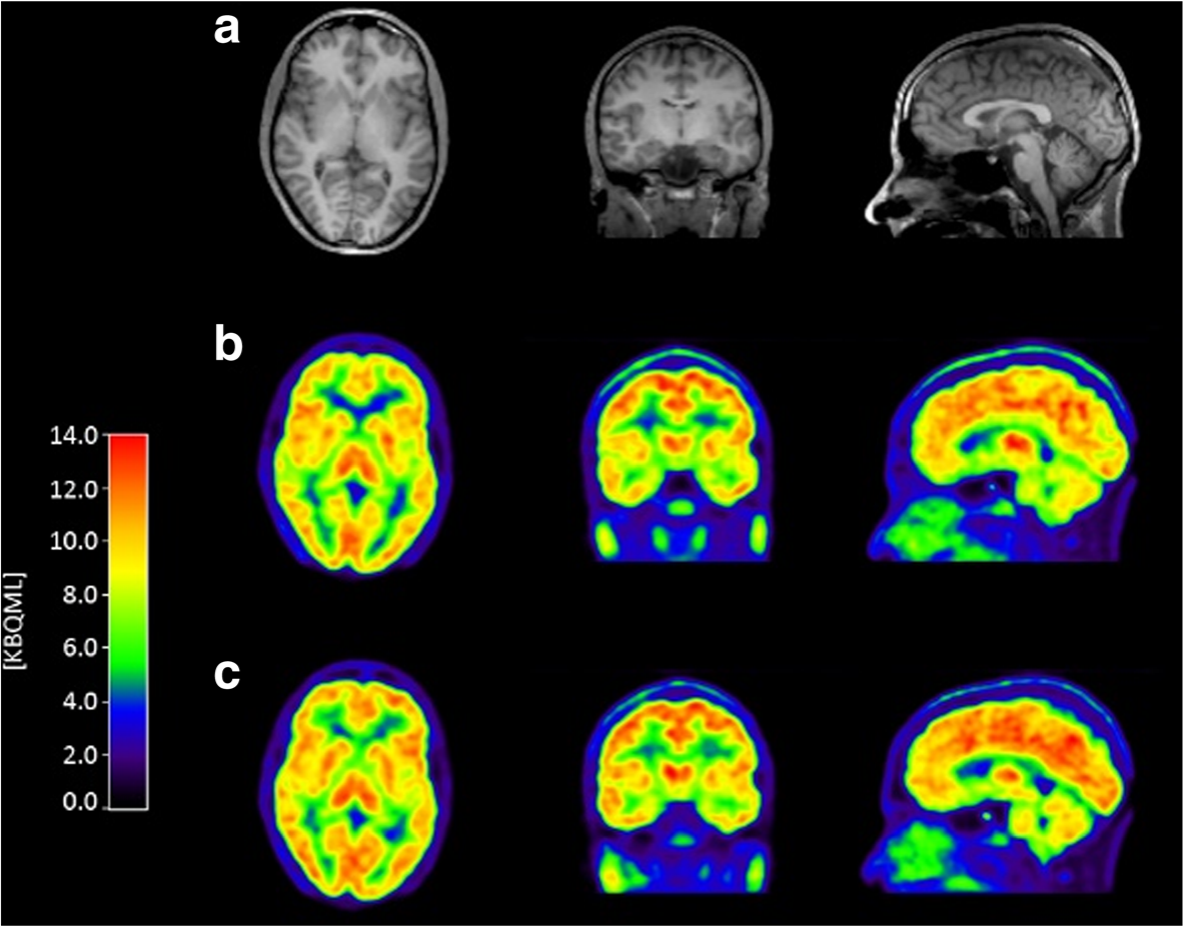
Transaxial, coronal, and sagittal views of MR images (a) and averaged PET images from 4.25–70 min for the test scan (b) and retest scan (c) of subject 1. Activity is expressed in kBq · mL^−1^
